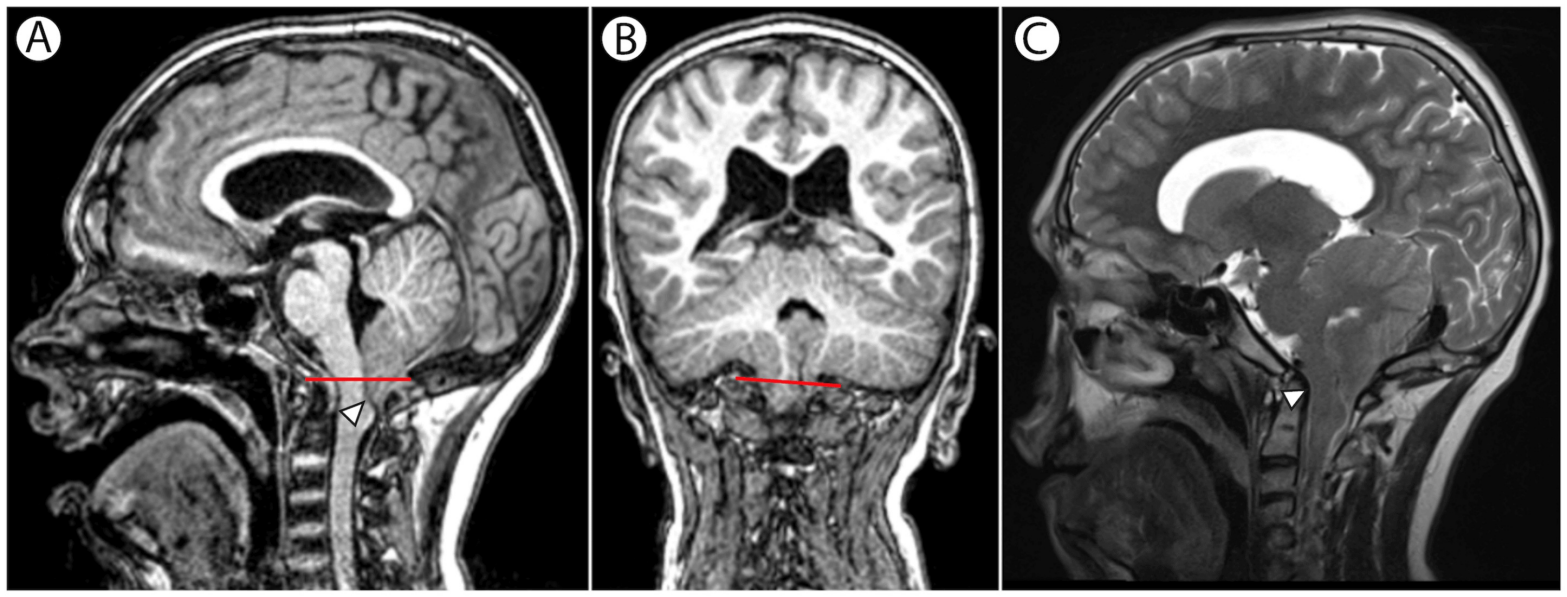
MRI of the brain A: 3D T1-MPRAGE sagittal reformat shows cerebellar tonsillar herniation below the foramen magnum, as indicated by McRae's line (red line); the posterior fossa appears hypoplastic, with caudal descent of the obex (arrowhead). B: 3D T1-MPRAGE coronal reformat highlights the extent of cerebellar tonsillar herniation (below the red line). C: Coronal T2-weighted image reveals CSF obliteration at the foramen magnum and ventral compression of the lower medulla (arrowhead), without edema or syringohydromyelia. T1-MPRAGE -  T1-weighted magnetization prepared rapid gradient echo; CSF - cerebrospinal fluid
